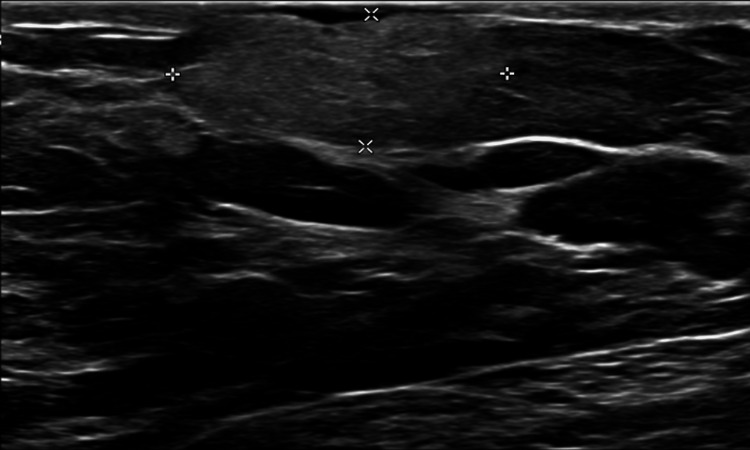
Gray-scale ultrasound image of the left breast upper outer quadrant palpable lump at the 2:00 axis, 8 cm from the nipple, demonstrates an indistinct, oval, parallel, hyperechoic mass. cm - centimeters Four (4) white measurements which highlight the axial and transverse measurements of the lesion.
